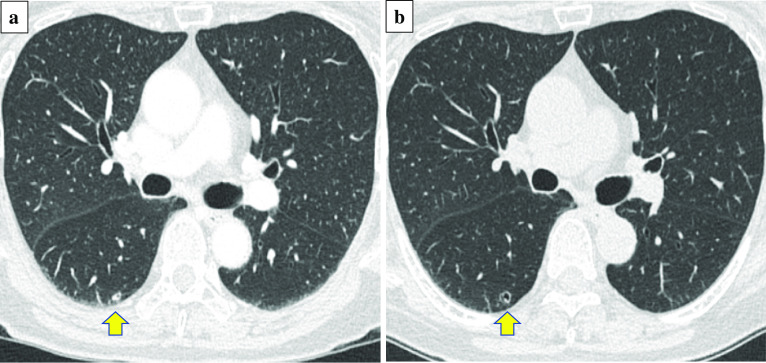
Serial CT findings of a ciliated muconodular papillary tumor (CMPT) at a colectomy performed 14 months prior and b thoracoscopic surgery. Yellow arrows indicate tumor growth from the nodule to a cavitary lesion suspicious of metastasis of colorectal cancer
